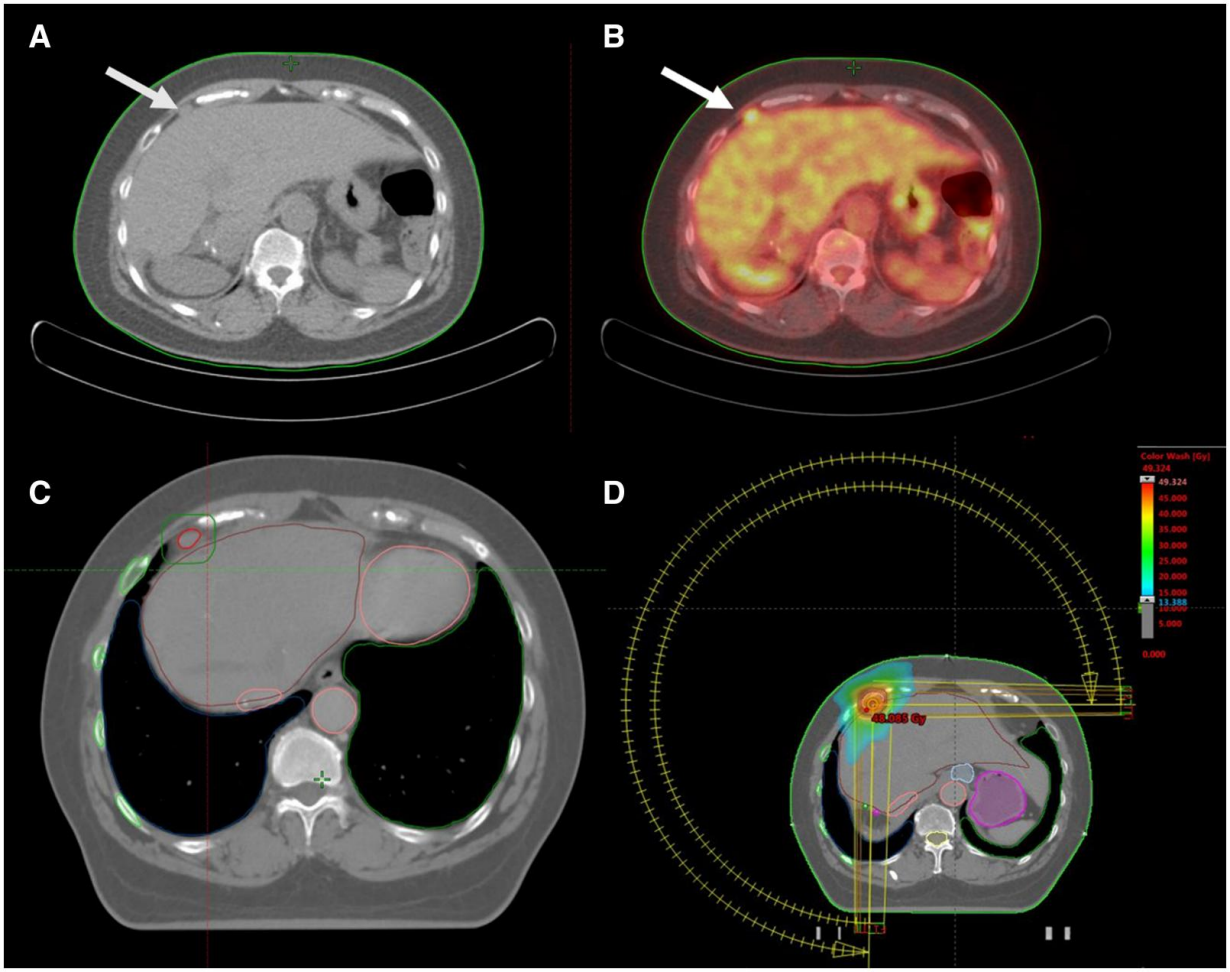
Peritoneal metastasis (hepatic dome) managed by SBRT: radiologic and dosimetric features. (A) Unenhanced CT image demonstrating peritoneal metastasis (arrow) regarding the hepatic dome. (B) ^18^F-fluorodeoxyglucose positron emission tomography/computed tomography (PET/CT) image showing tracer uptake of the peritoneal metastasis. (C) Unenhanced CT-scan with the gross tumour volume (GTV) encompassing the lesion. The planning target volume (PTV) covered the GTV with 5 mm margin. The treatment was performed with 6 megavoltage (MV) flattening filter free (FFF) photons on a linear accelerator using VMAT technique. (D) VMAT plan showing axial images of radiotherapy dose colour wash, with a hot spot (48.085 Gy) within the PTV.
